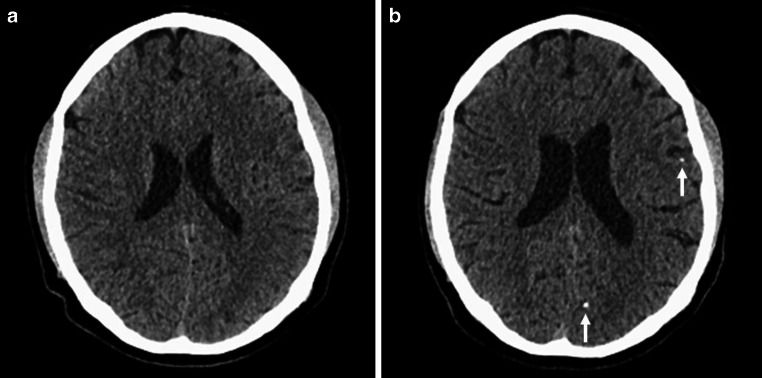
Unenhanced brain CT scan at discharge after the first treatment (a) and on admission for the second stroke (b). CT scan after carotid artery stenting and stent fracture shows hyperdense stent fragments in the left frontal lobe and the parietooccipital fissure via fetal origin of the posterior cerebral artery (white arrows in b) which were not present before stenting (a)
